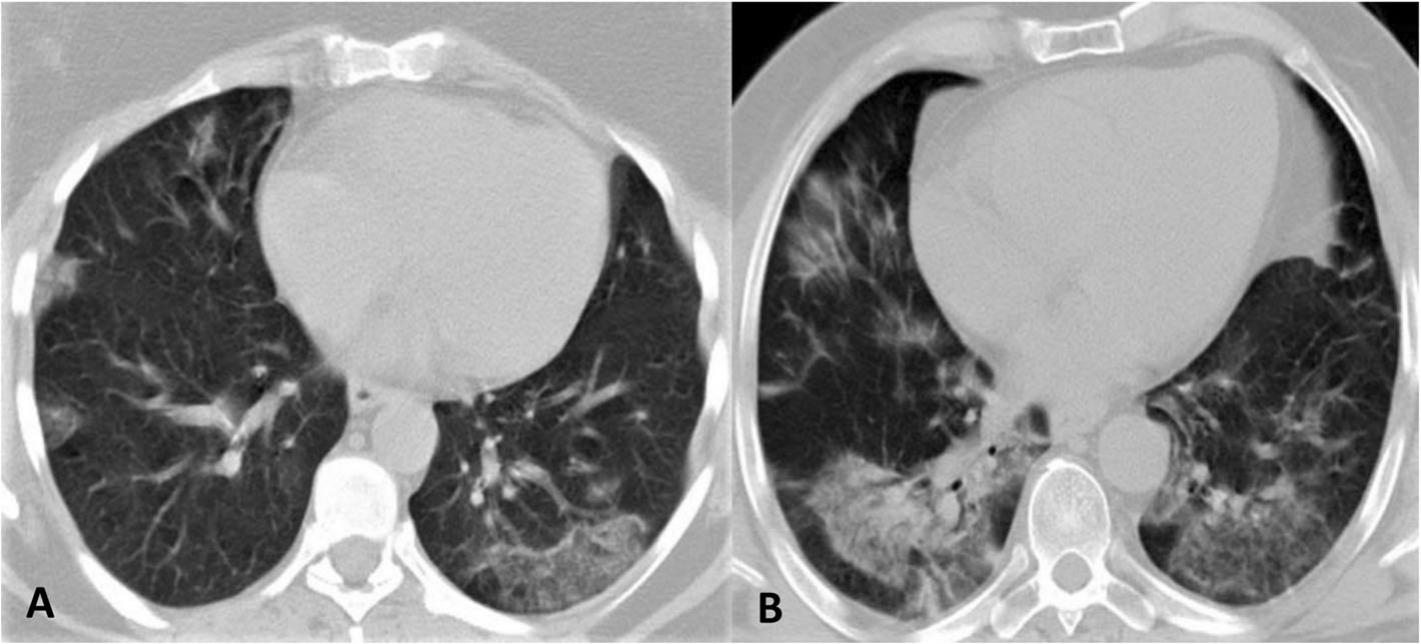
CT images of asymptomatic patients. a Axial CT image of a 50-year-old RT-PCR (+) asymptomatic female patient shows bilateral peripheral ground glass areas. b Axial CT image of a 52-year-old RT-PCR (−) asymptomatic male patient shows bilateral peripheral ground glass opacities, consolidation, and reticular opacities
